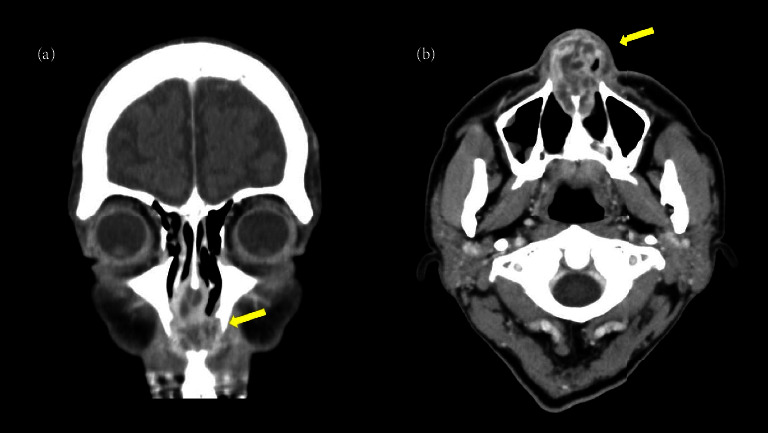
(a). Coronal contrast-enhanced CT of the paranasal sinuses showing multifocal abscess in the nasal septum (yellow arrow). (b). Axial contrast-enhanced CT of the paranasal sinuses showing multifocal abscess in the nasal septum (yellow arrow).
